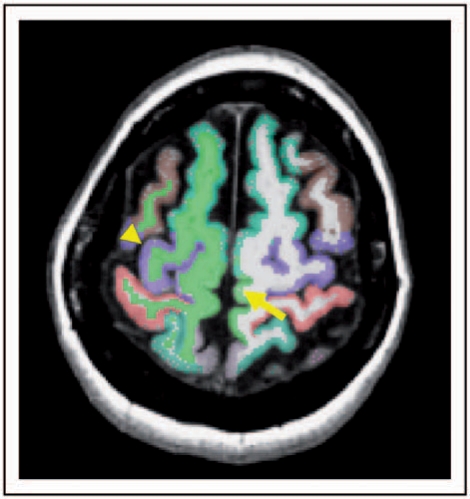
Results of the cortical parcellation overlaid onto the T1-weighted scans of the same patient as in Figures 1 and 2. The arrowhead indicates the R precentral cortex and the arrow indicates the L paracentral cortex (*z* = 58).
